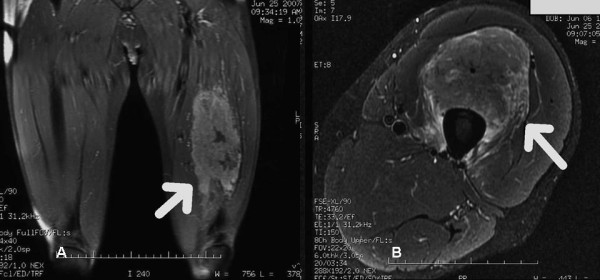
A and B: Magnetic Resonance Images of left thigh.
